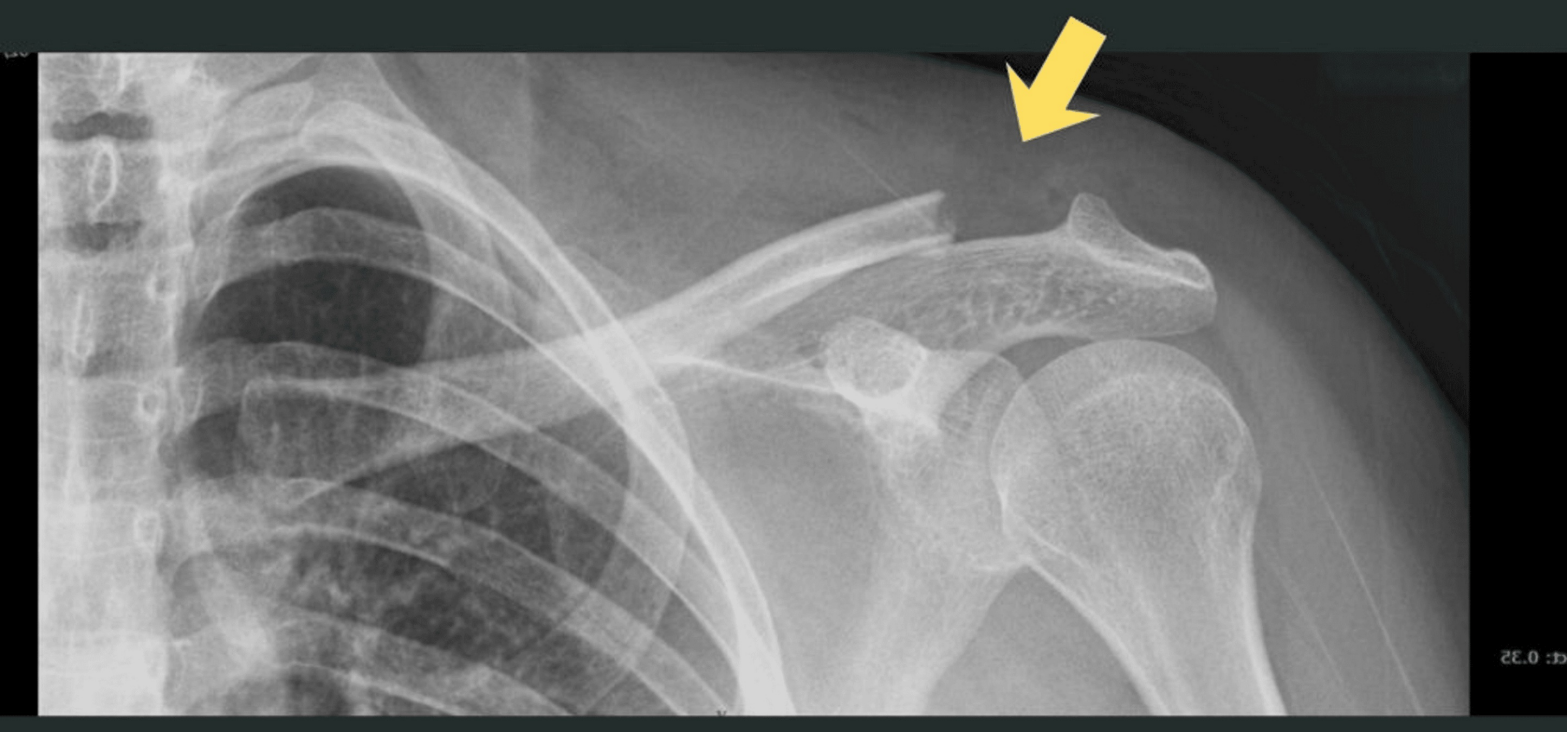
Post-operative X-ray of left clavicle. Post-operative X-ray of the left clavicle in AP view showed no superior migration of the lateral distal clavicle (yellow arrow).
